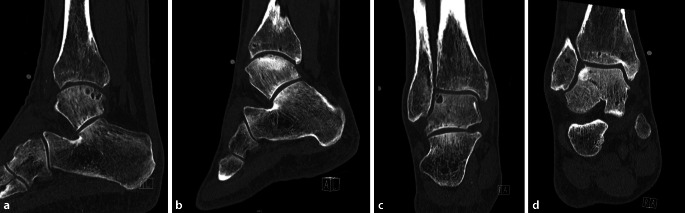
Preoperative and postoperative (1-year follow-up) CT scans of the same patient. a Sagittal preoperative CT scan, b sagittal postoperative CT scan, c coronal preoperative CT scan, d coronal postoperative CTscan
